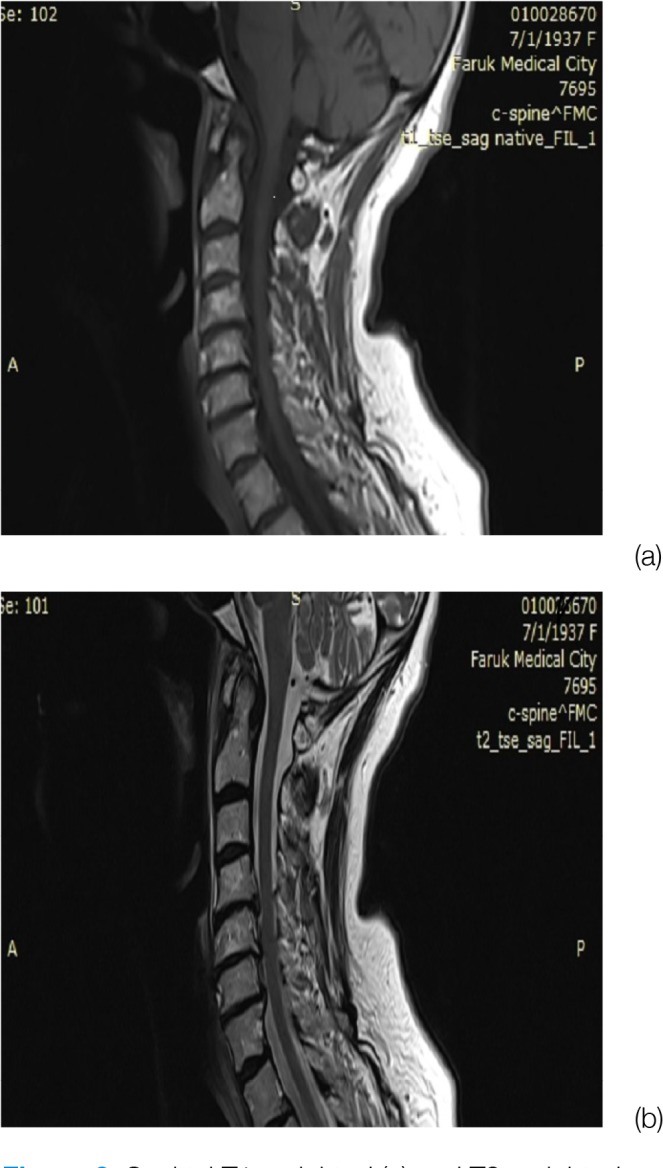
Sagittal T1-weighted (a) and T2-weighted (b) Cervical MRI: soft tissue thickening posterior to the dens.
